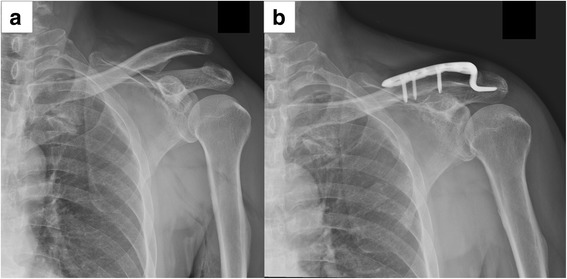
Coracoclavicular fixation with hook plate for a type V AC dislocation after motorcycle accident injury. a Preoperative plain radiograph. b 1-month postoperative plain radiograph
